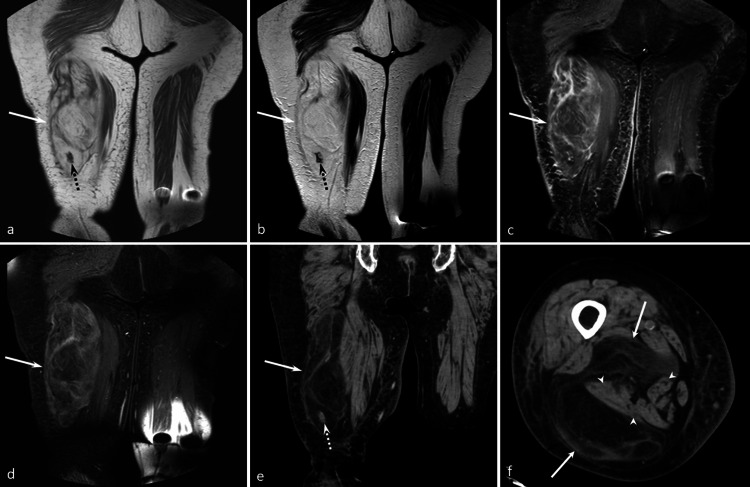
An 87-year-old woman with an ASCPLT in the right thigh Coronal T1WI (a), coronal T2WI (b), coronal FST2WI (c), and coronal FSCET1WI (d) demonstrate a well-defined intermuscular fatty mass (arrows) containing non-fatty components (dotted arrows). Coronal CT (e) shows a well-defined intermuscular fatty mass (arrow) with non-fatty components (dotted arrow). Axial CT (f) shows well-defined intermuscular fatty mass (arrow) with compressed hamstring muscles. The imaging findings are consistent with a spindle cell lipoma pattern.
